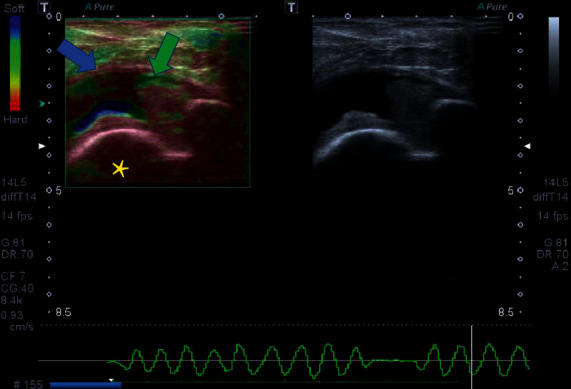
A 34-year-old patient with silicone implants for 5 years. Breast ultrasonography shows thickening of the breast implant capsule, associated with a granuloma in focal areas (green arrow). Intracapsular collection and intracapsular mass are also observed (blue arrow). The fluid collection is soft at elastography. The yellow asterisk shows the breast implant.
